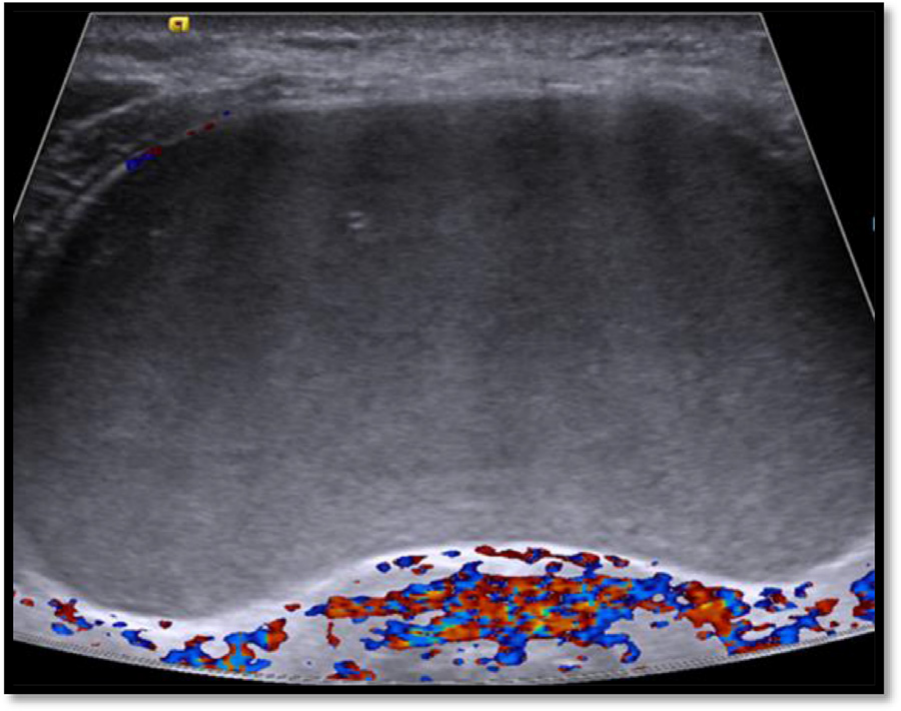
Doppler ultrasound showing avascular cyst.
